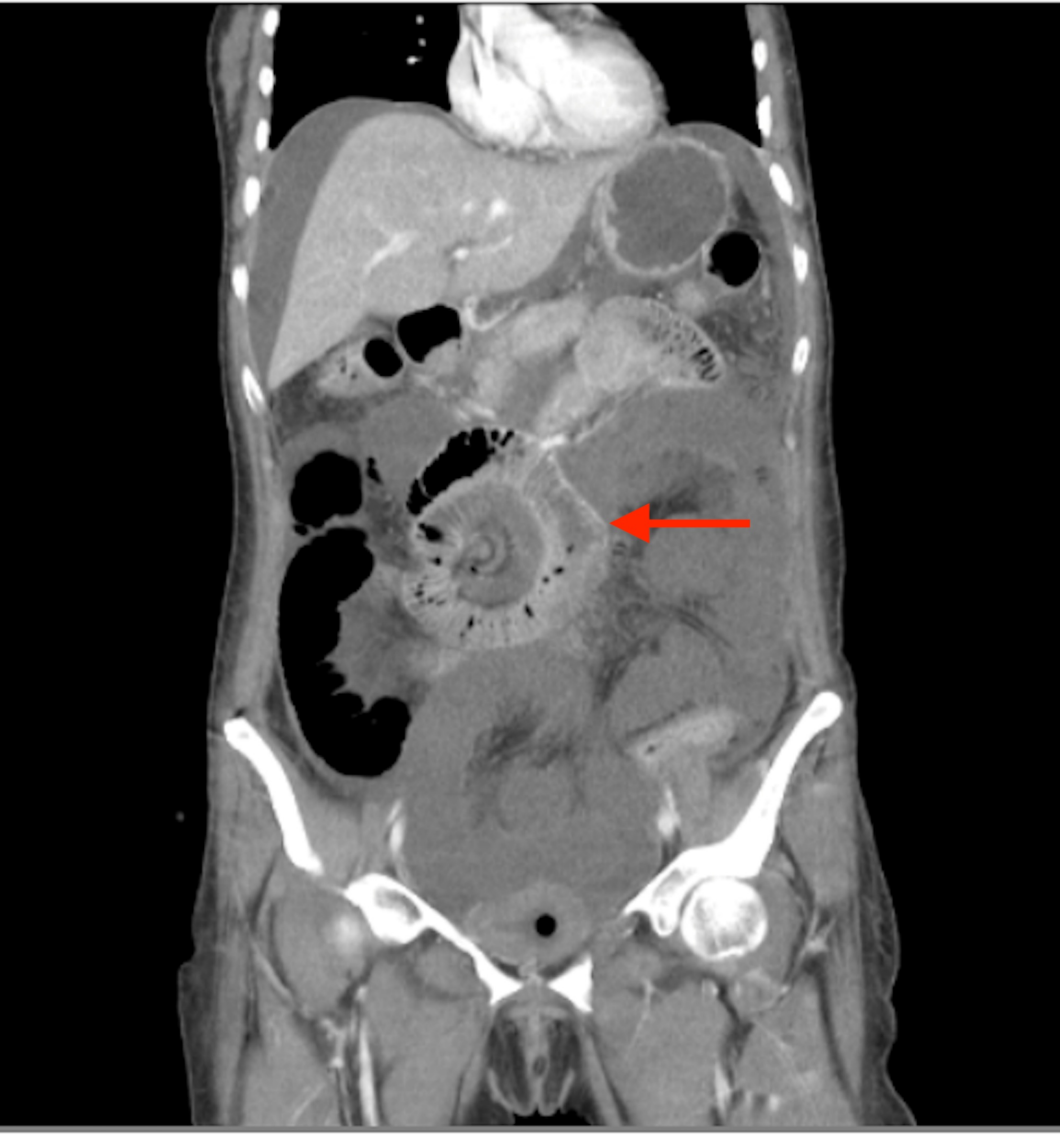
Computed tomography scan of the patient showing the classic “whirlpool” sign (red arrow).
